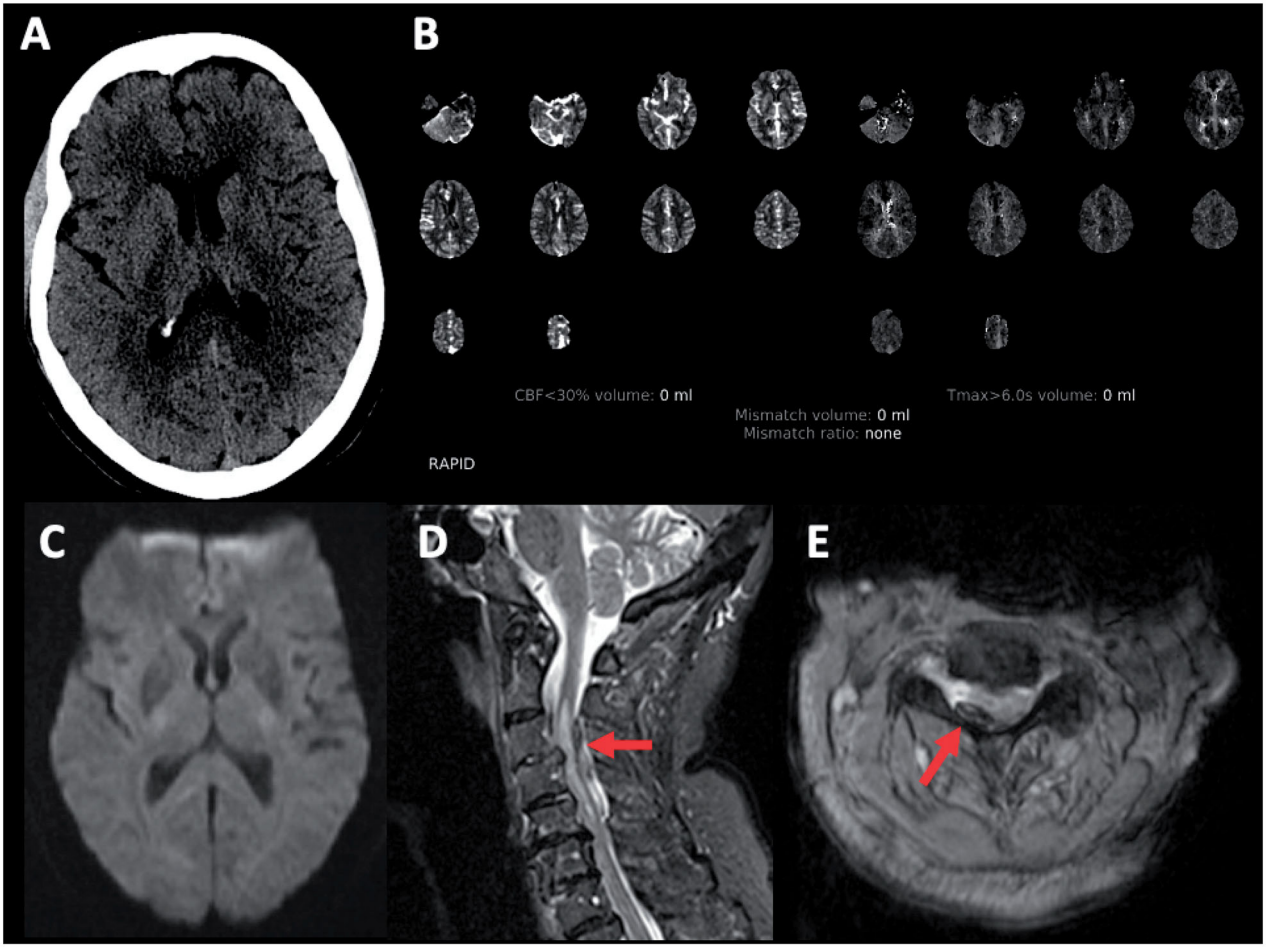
80-year-old female with atrial fibrillation on apixaban. She presented with a 90-min history of sudden onset right arm and leg weakness sparing the face with normal sensation. A,B: Axial computed tomography (CT) head, CT angiogram (not included) and CT perfusion were unremarkable. Tissue plasminogen activator (tPA) was no given because the patient is on an anticoagulant. C: Diffusion-weighted magnetic resonance imaging (DWI-MRI) was normal. D,E: Sagittal and axial MRI spine revealed right posterolateral epidural haematoma, a multifocal severe spinal canal stenosis, and spinal cord oedema.
